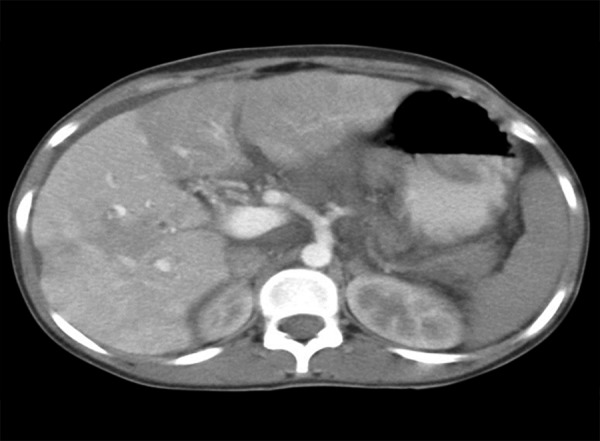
Computed tomography scan shows an enlarged portal vein with multiple linear filling defects extending into the main portal vein
